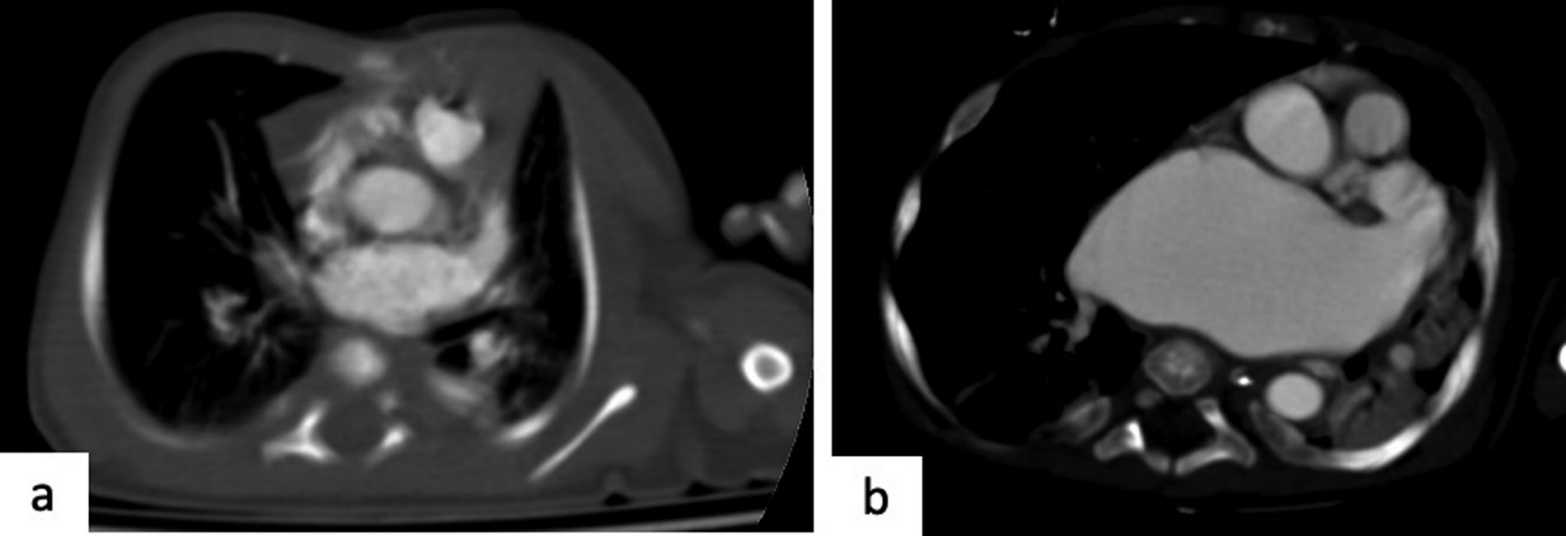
Progressive left atrial dilatation. Axial computed tomography (CT) image showing progressive expansion of the left atrium 2 months later.
